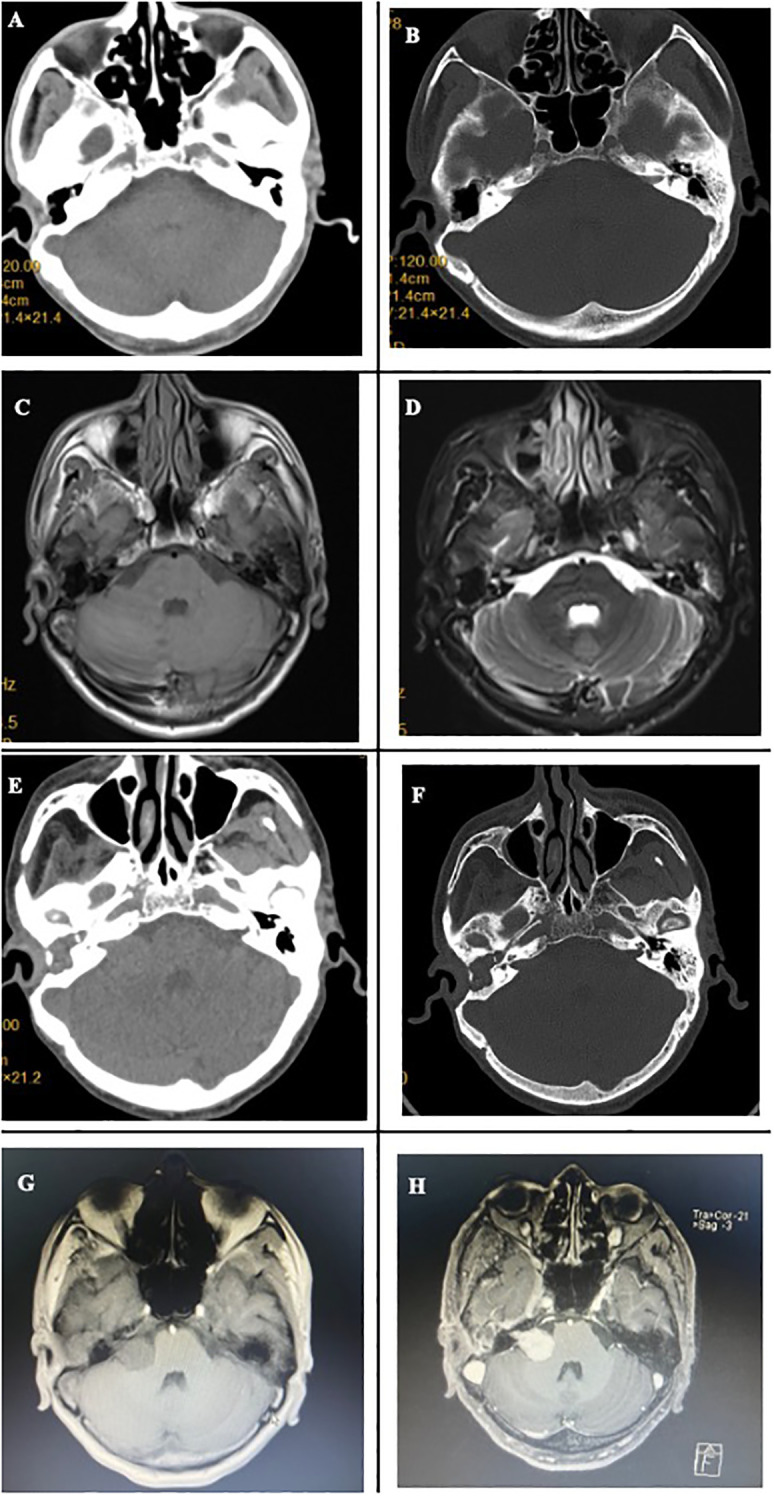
Imaging findings from 2019 and Preoperative imaging findings before the second surgery. (A, B) Noncontrast head CT and bone window show no tumor recurrence or metastasis in the intracranial region or right EAC. (C, D) Head MRI reveals no evidence of tumor recurrence or metastasis in the intracranial region or right EAC. (E) Noncontrast head CT shows an isodense mass in the right CPA. (F) CT bone window demonstrates significant widening of the right IAC. (G) Noncontrast head MRI reveals a slightly hypointense T1 signal mass in the right CPA. (H) Contrast-enhanced head MRI shows marked homogeneous enhancement of the right CPA mass, with tumor growth extending into the IAC.
